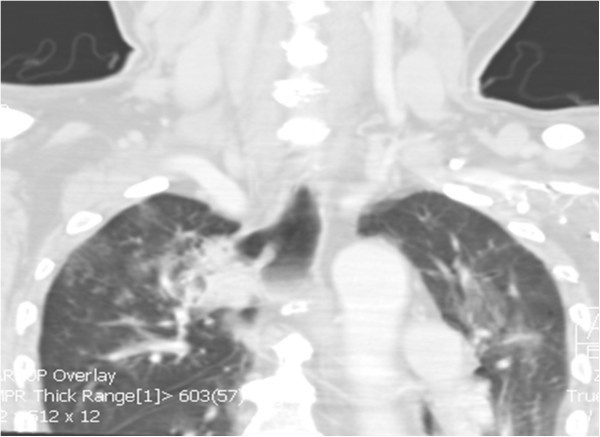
CT scan of the chest was suggestive of an esophageal diverticulum communicating with apical segment of right upper lobe.
